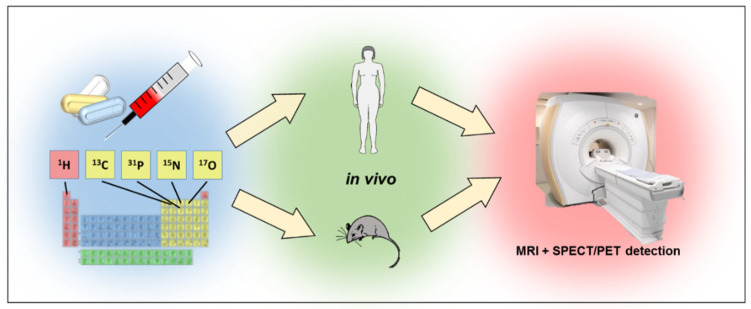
Using MRI to trace pharmaceuticals with different elements.
